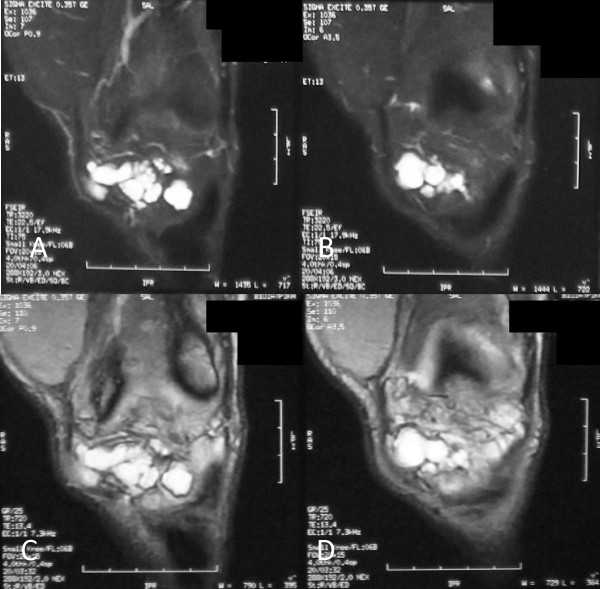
Coronal magnetic resonance images of the anterior aspect of the knee. (A, B) Sequential T2-weighted images and (C, D) sequential proton density (PD) images of a multilobulated mass with high signal intensity.
